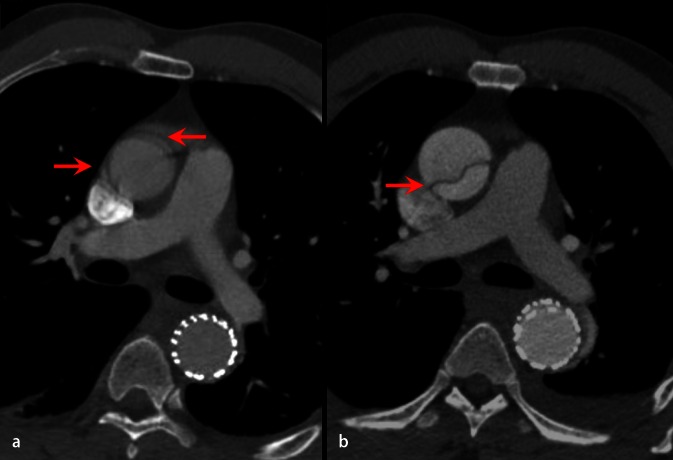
Computed tomography images acquired in the same imaging plane in a patient presenting with chest pain who had previously undergone endovascular repair of the descending thoracic aorta. Image a was acquired at presentation and image b 48 h later. The findings in image a (*arrows*) could represent artefacts or a new aortic dissection. At 48 h (image b) a clear dissection is seen in the ascending aorta (image b
